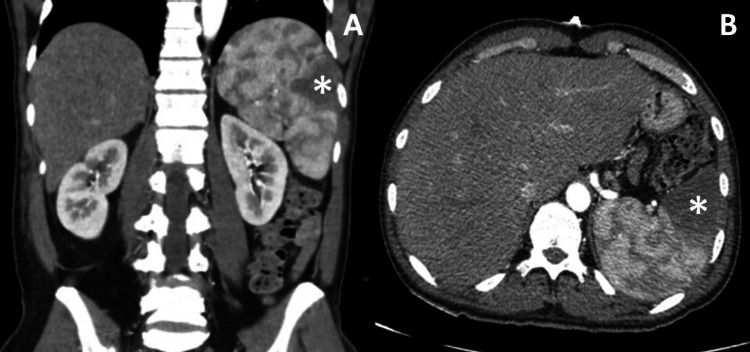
Abdominopelvic CT scan in the (A) coronal and (B) axial views showing extensive splenic infarctions (asterisks)
